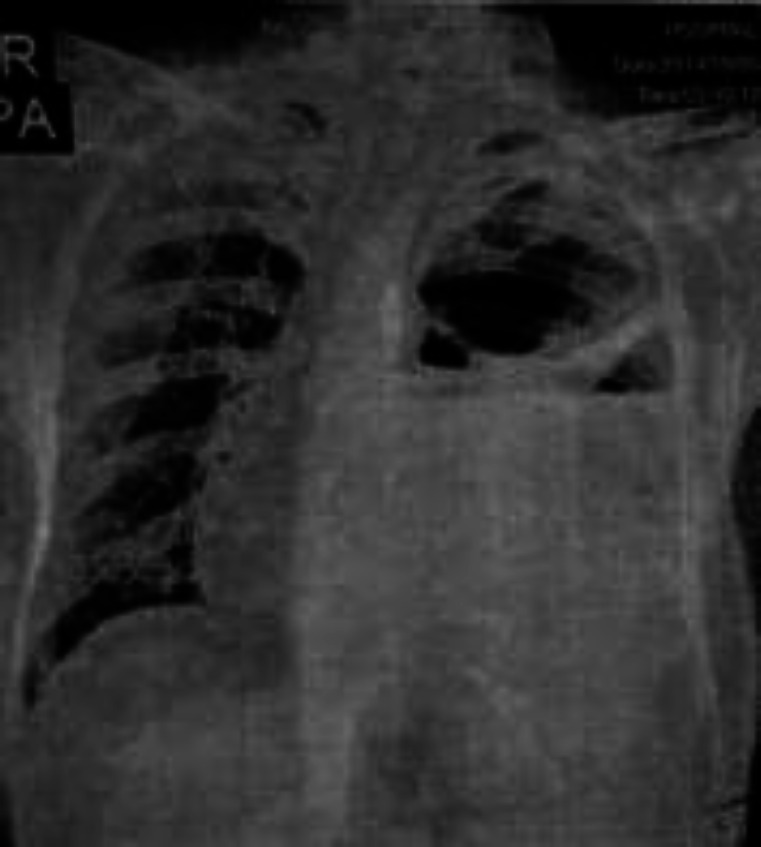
Chest radiograph before laparatomy and chest tube drainage revealing left sided hydropneumothorax
